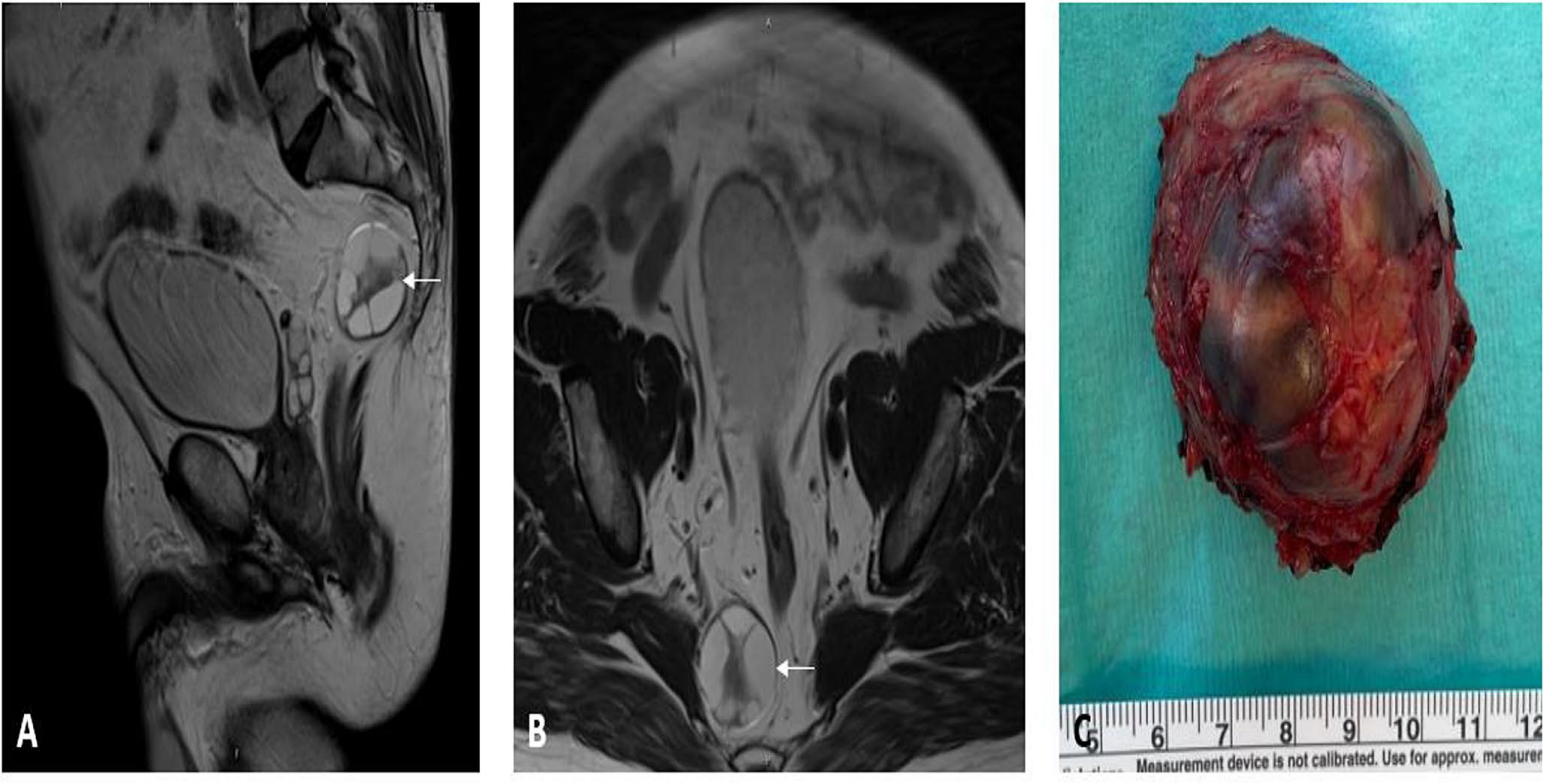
58-year-old patient with a retrorectal schwannoma on preoperative MRI:  sagittal T2 (A),  axial T2 (B), and the postoperative macroscopic view of the schwannoma (C)
